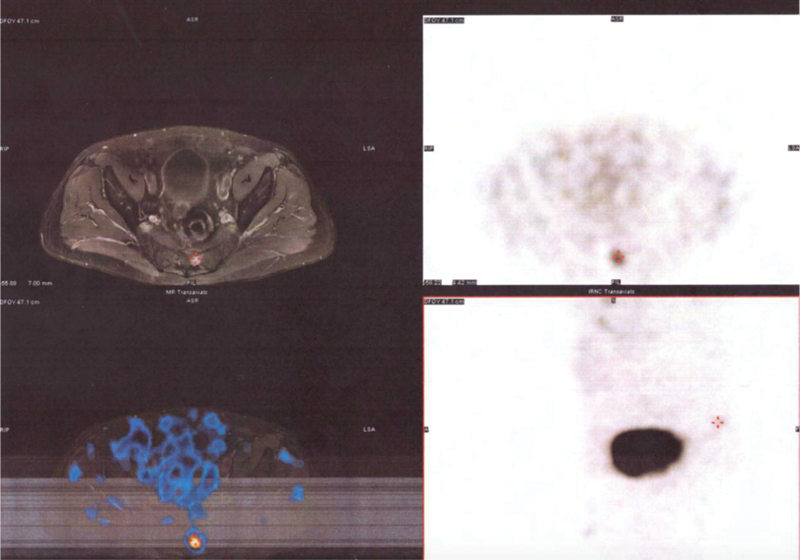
SPECT-CT (Single-photon emission computed tomography) with 99mTc-Tektrotyd: small intake in the area of the resection (
